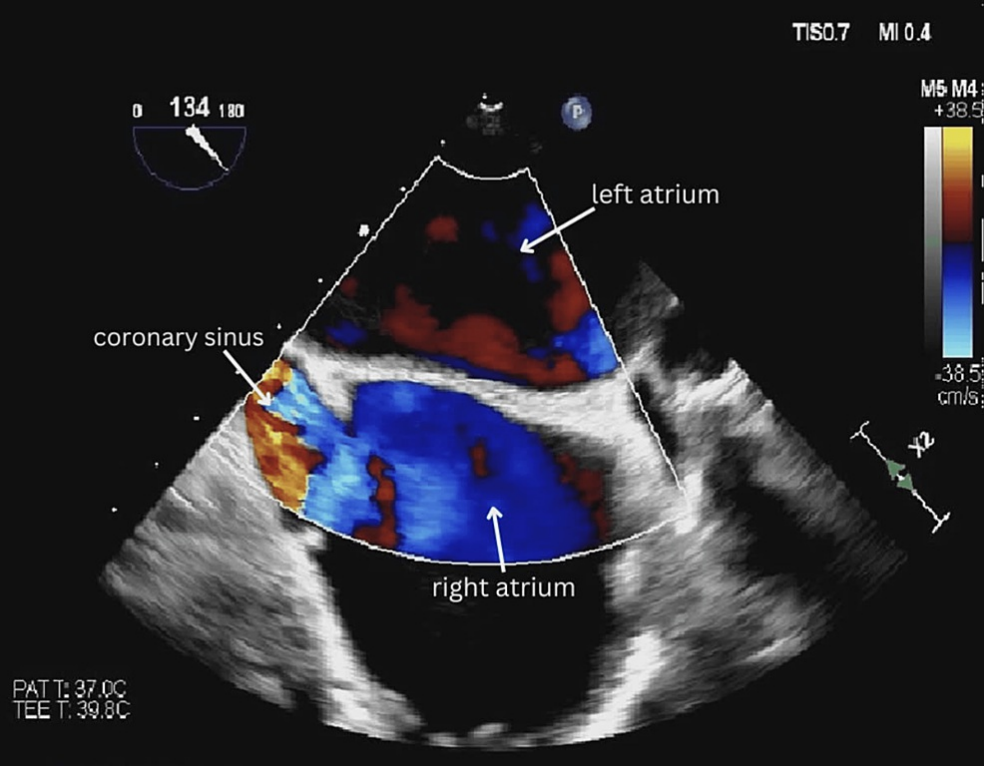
Transesophageal echocardiogram mid-esophageal bicaval view showing systolic flow acceleration from the coronary sinus into the right atrium
